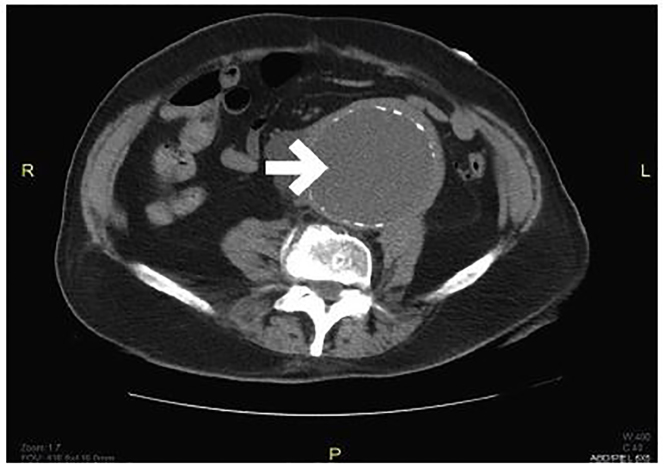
Computed tomography without contrast of the abdomen and pelvis in axial view with an arrow demonstrating a 9 × 9 cm infrarenal aortic aneurysm.
